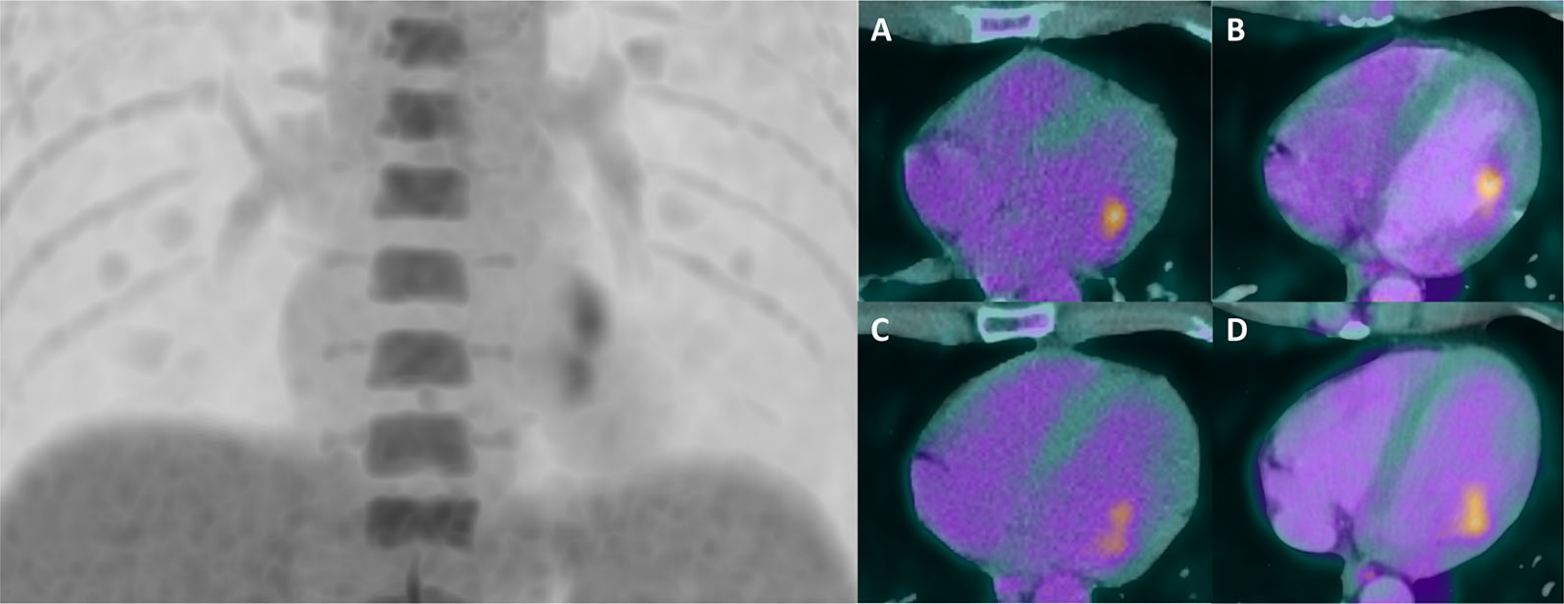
PET/CT and computed tomography angiography (CTA) findings. To assess for presence of extracardiac septic foci, a ^18^F-FDG PET/CT, performed after 3 days of dietary preparation and heparin pre-administration to suppress physiological myocardial FDG uptake was performed. Left, Maximum intensity projection image of the thorax showing pathological accumulation of ^18^F-FDG in the heart area. Right, A, B Transaxial view of ^18^F-FDG PET/CT (A) and ^18^F-FDG PET/CTA (B) showing focal FDG uptake of the chordea tendinae at the insertion in the anterolateral papillary muscle. C, D Transaxial view of ^18^F-FDG PET/CT (C) and ^18^F-FDG PET/CTA (D) showing focal FDG uptake of the chordea tendinae between the valve leaflet and the posteromedial papillary muscle. Due to the poor sensitivity, a negative ^18^F-FDG PET/CT cannot rule-out NVE. Based on its excellent specificity, positive valvular- or peri-valvular FDG uptake could be used to rule-in NVE, since no physiological FDG-uptake is expected in this area.^1^ When using ^18^F-FDG PET/CT as major criterion for the NVE diagnosis, with state-of-the art dietary patient preparation, contemporary PET/CT devices (better spatial resolution) as well as delayed cardiac acquisitions (better contrast resolution), the sensitivity of ^18^F-FDG PET/CT in the NVE diagnosis is increased up to 80%, without altering the excellent specificity.^2^ In NVE, valve leaflets and peri-annular area are the most commonly sites of cardiac FDG-uptake. Isolated involvement of the submitral apparatus in absence of myocardial FDG uptake are very rare, evoking inflammatory cardiomyopathy (sarcoidosis), intraventricular thrombus, neoplasm or infective endocarditis^3^
